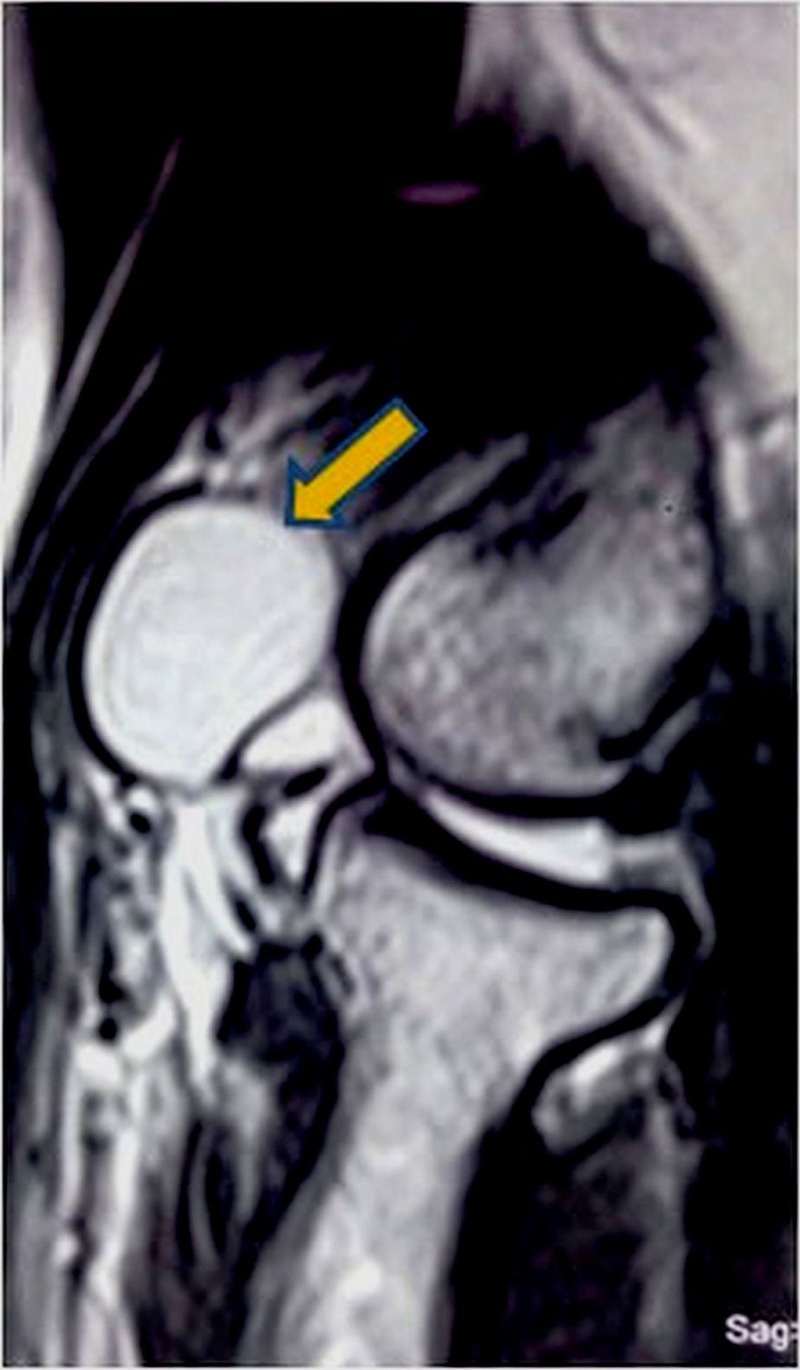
MRI T2 weighted saggital view showing hyerintense cyst anterior to radiocapitellar joint
